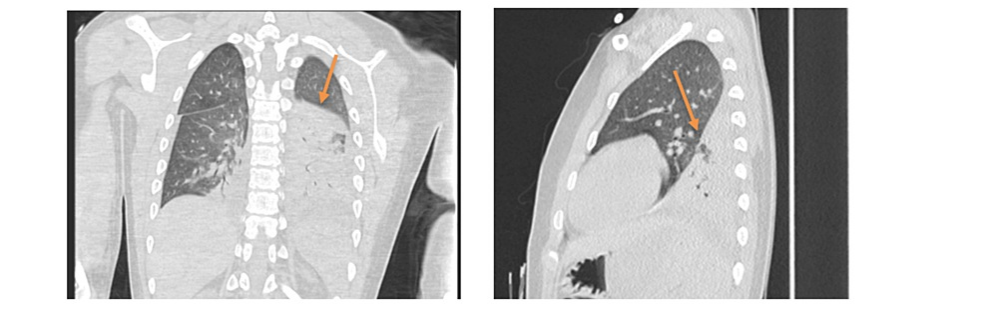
High-resolution computed tomography (HRCT) coronal and sagittal views on day 10 of hospital admission Both lungs show diffuse ground-glass density. Collapse-consolidation is seen in the right posterior basal segment and left lower lobe with an air-bronchogram. Small effusions are present bilaterally.
